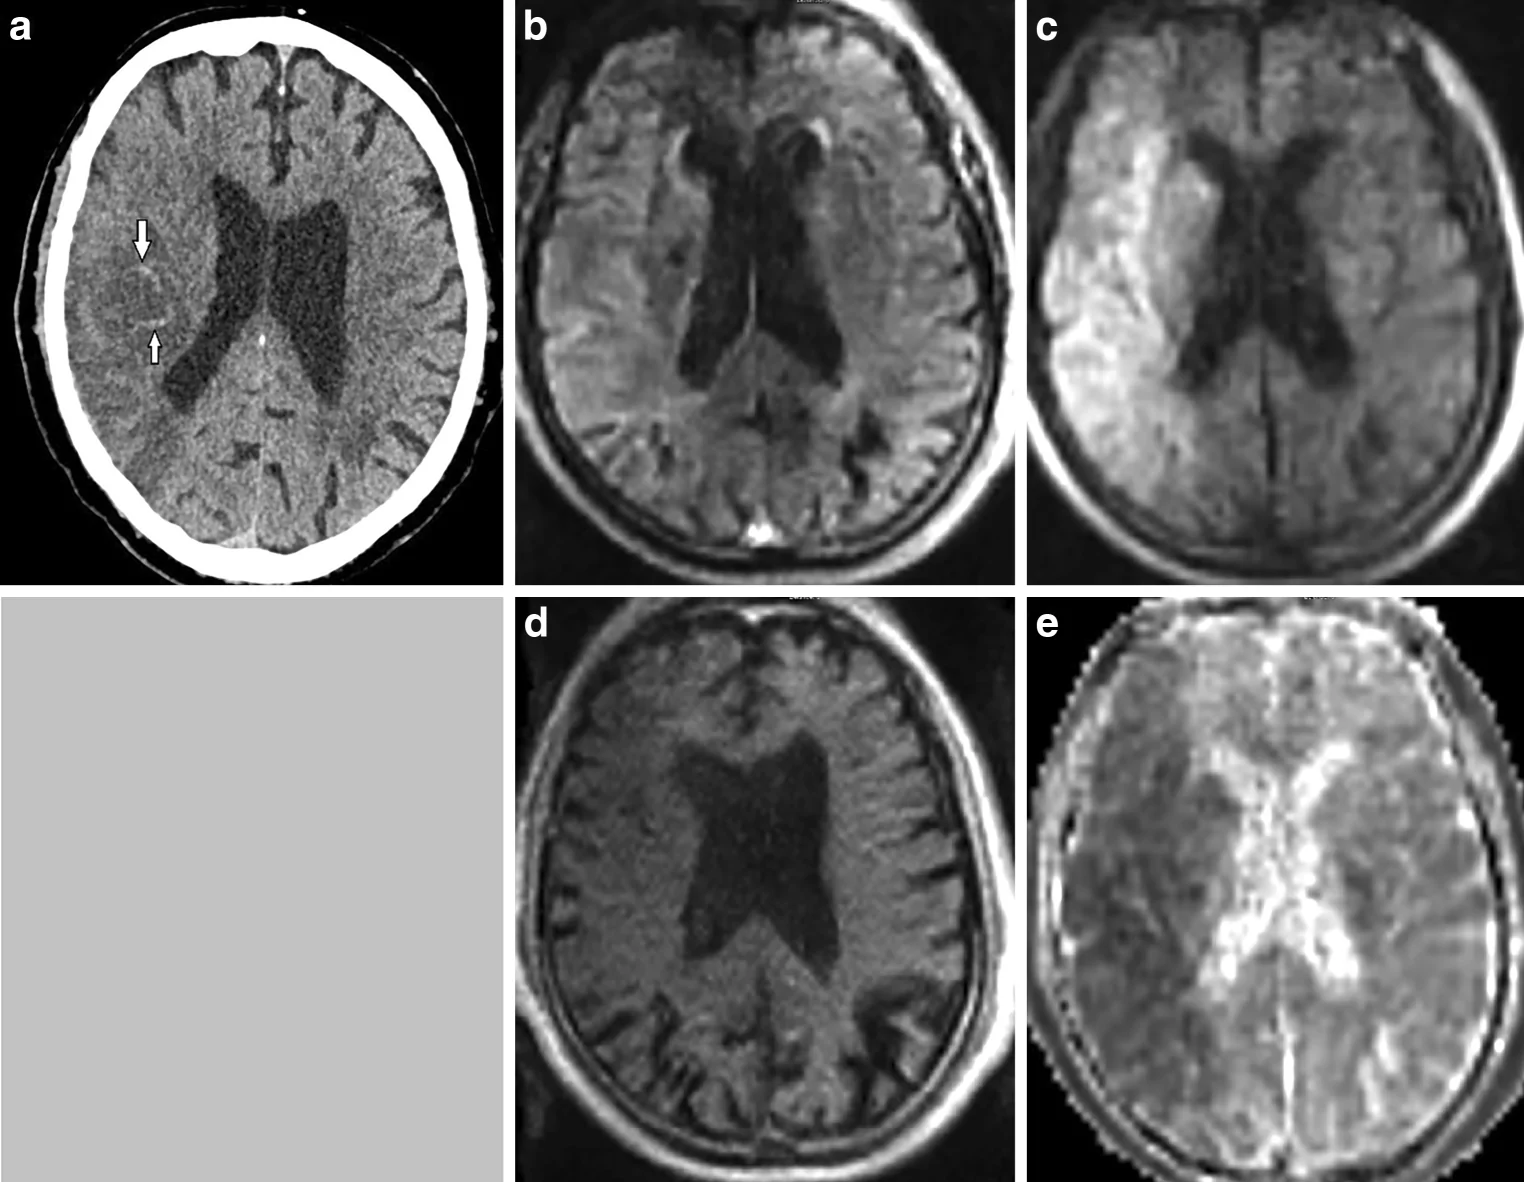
Hemorrhagic transformation (HBC1a) within the extensive subacute left-sided middle cerebral artery territory infarction was detected on CT (arrows in a) but was not visible on ULF-pMRI (b–e)
